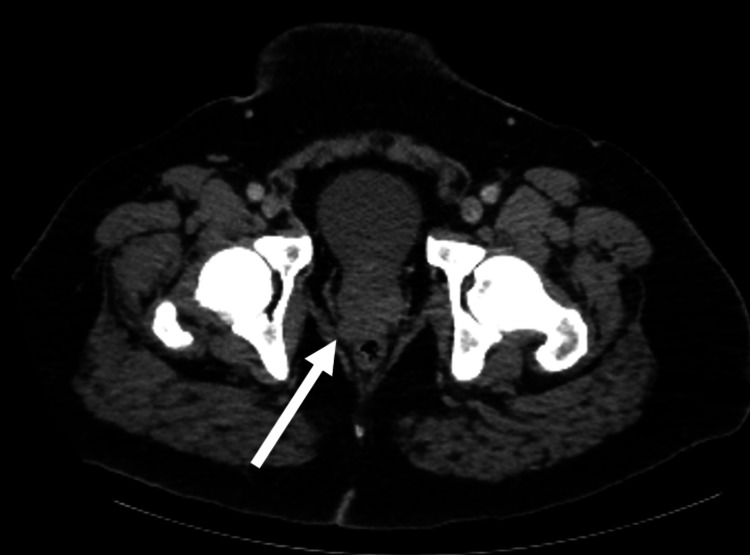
Axial CT scan of pelvic region There is mild bulging along the right posterior prostate base and seminal vesicle (white arrow) which may reflect the primary tumor.
